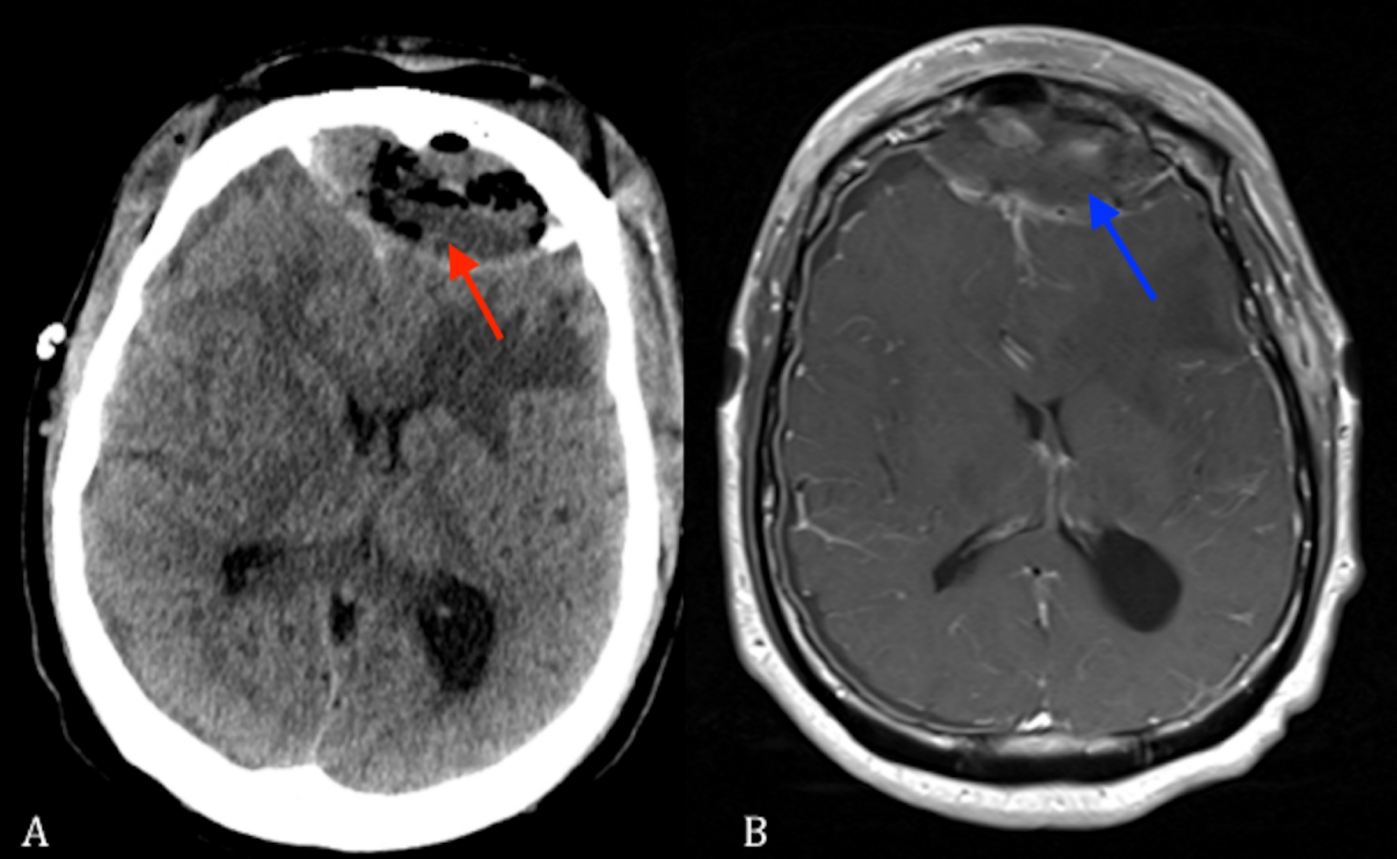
(A) Axial non-contrast CT scan showing a frontal fluid collection under the craniotomy bone flap (red arrow). (B) Axial T1 MRI with contrast showing a frontal fluid collection under the craniotomy bone flap (blue arrow). CT: Computed tomography; MRI: Magnetic resonance imaging.
